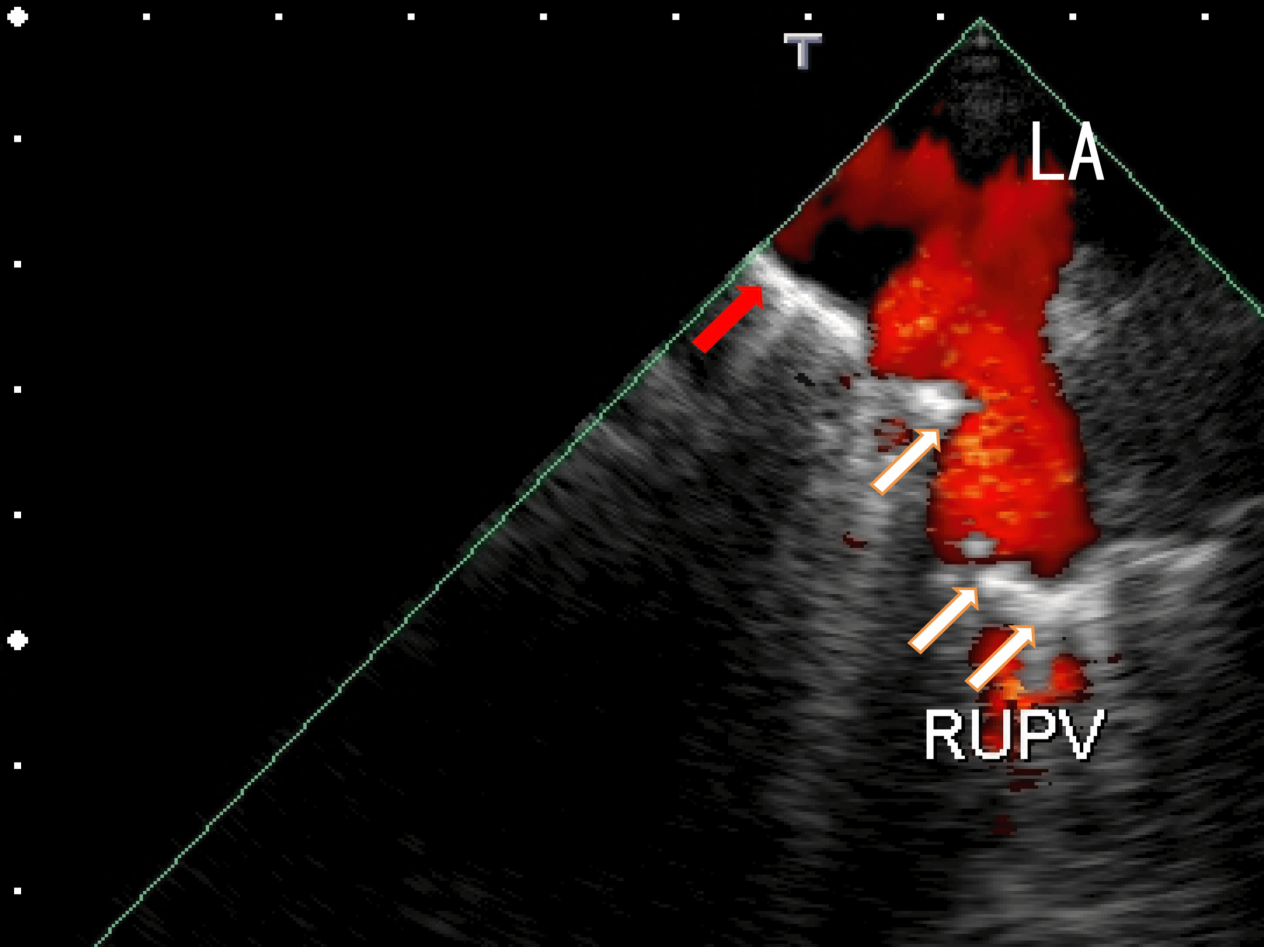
TEE images showing thrombi TEE images showing thrombi in the RUPV (white arrows) and the LA (red arrow). The LA thrombi extend from the right lower pulmonary vein (RLPV) thrombi and appear as a white line parallel to the direction of the RLPV. The size of the hyperechoic region is approximately 3 mm × 15 mm. The other several thrombi are attached to the wall of the RUPV and present as hyperechoic regions. These hyperechoic regions measure approximately 3 mm × 7 mm and are perpendicular to the direction of the RUPV. Some parts of the thrombi have white shadows, indicating areas of calcification. Some of the white areas are surrounded by dark areas that connect to other thrombi and are attached to the LA wall. TEE: transesophageal echocardiography, LA: left atrium, RUPV: right upper pulmonary vein
